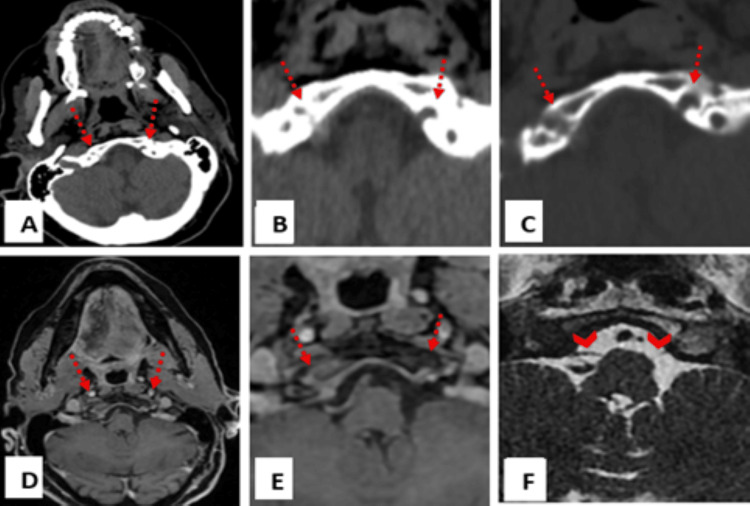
(A) Axial CT scan, (B) with magnified view: an intracranial extra-axial lesion at the level of the cisternal segment of the hypoglossal nerve with slight extension into the right hypoglossal canal. (C) Magnified view, axial CT scan bone window: cortical bone erosion of the right hypoglossal canal (right dashed arrow) compared to the intact canal on the left side (left dashed arrow). (D) T1 fat-saturated MR imaging with contrast administration, (E) with magnified view: 6 x 13 mm extra-axial enhancing lesion with a “dural tail” sign at the level of the cisternal segment of the right hypoglossal nerve extending to the right hypoglossal canal, with overlying bone edema and enhancement. (F) Magnified view, axial FIESTA MR imaging: thickening of the cisternal segment of the right hypoglossal nerve (right arrow head) compared to the intact and thin nerve on the left side (left arrow head). FIESTA MR: fast imaging employing steady-state acquisition magnetic resonance
